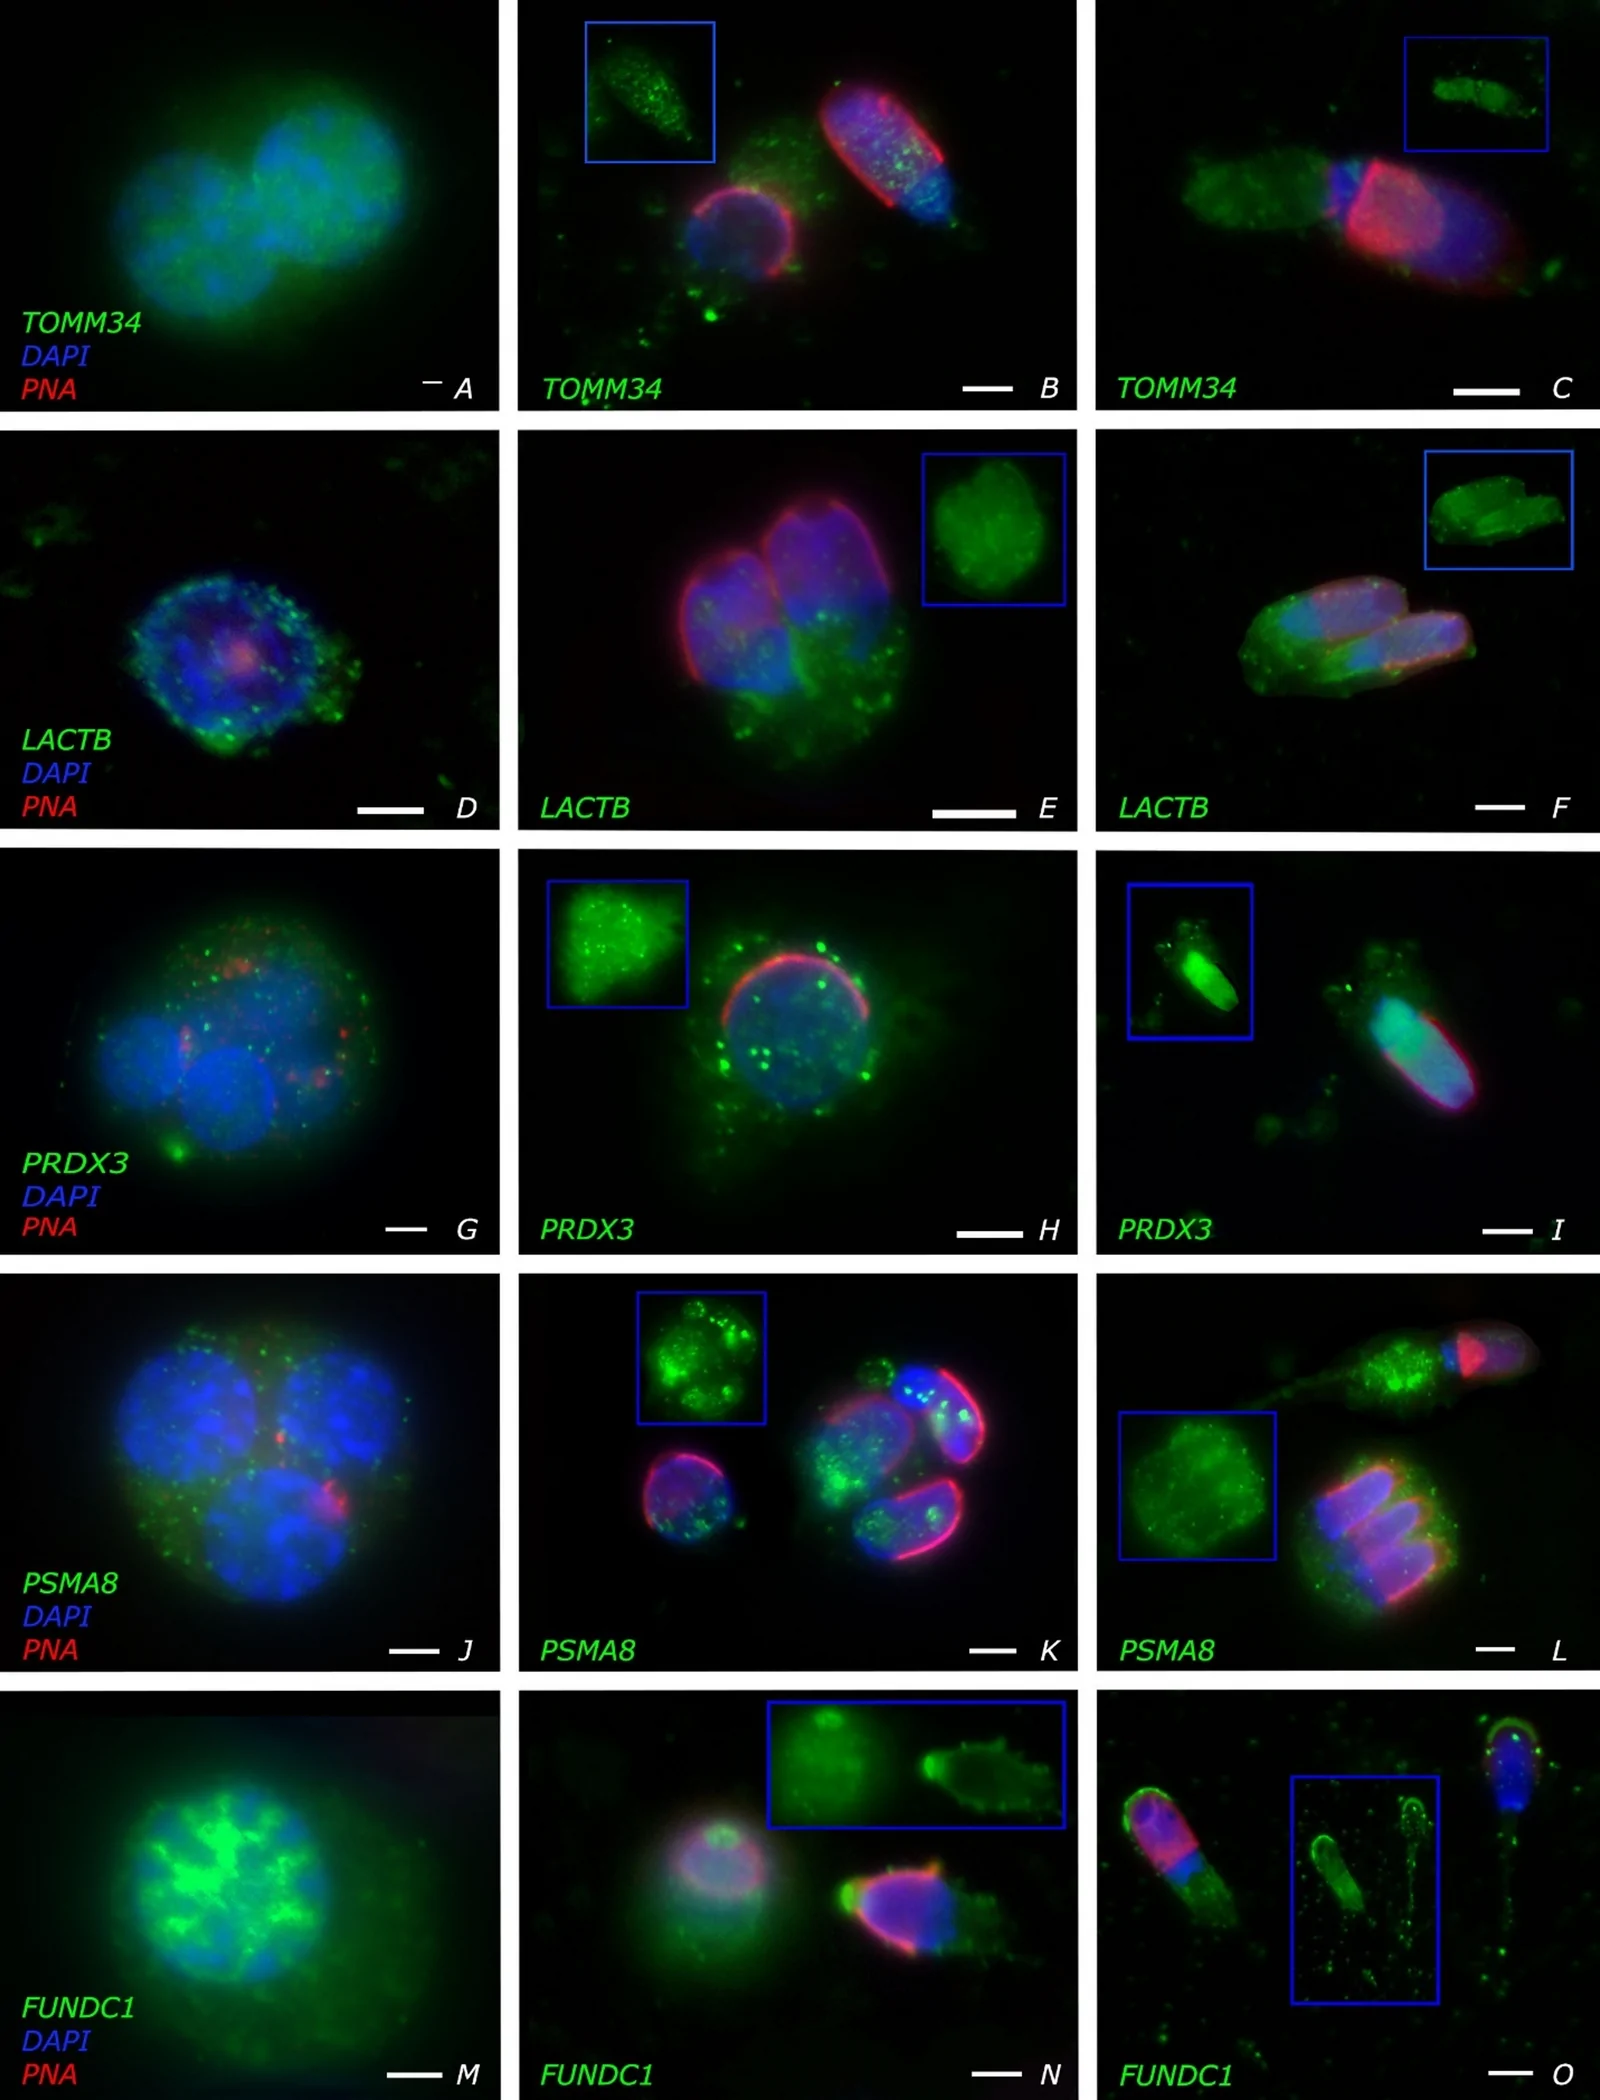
Immunocytochemistry of testicular germ cells provided visualization of the target proteins at various stages of spermatogenesis and steps of spermiogenesis. A The TOMM34 protein (green) was visualized within the cytoplasm of spermatocytes. B The signal persisted in the cytoplasm of round and early elongating spermatids, localizing faintly with the post-acrosomal region and developing connecting piece or centriole, as visible in the inset showing the TOMM34 signal alone. C As the spermatid elongation advanced, TOMM34 became weaker near the apical region, while stronger over the post-acrosomal region and cytoplasmic lobe/droplet and still present in the head. D The LACTB protein (green) was visualized within the cytoplasm of the spermatocytes. E The protein signal remained within the developing head and cytoplasm of the round spermatids. F In elongating spermatids, signals were localized to the outer acrosomal membrane, manchette, and the cytoplasmic lobe, as visible in the inset. G The PRDX3 protein (green) was visualized within the cytoplasm of the spermatocytes. H The signal intensified throughout the cytoplasm with the addition of granules/aggregates in round spermatids. I Through the elongation stages, PRDX3 can be seen in the head, with a stronger presentation in the post-acrosomal region. Protein signal decreased within the cytoplasmic lobes, as shown in the inset. J The PSMA8 protein (green) was visualized within the cytoplasm of the spermatocytes. The signal remained throughout the cytoplasm of round spermatids with the addition of granules. K There was a localization to the acrosomal membrane and post-acrosomal region. The PSMA8 can be visualized in the cytoplasm of elongating spermatids, with stronger signal on the outer edge of the acrosome (K, L). L In late-stage elongation and immature spermatozoa, there was a signal in the cytoplasm with an increase in signal intensity in the lobe/droplet and the developing mitochondrial sheath of the nascent tail. M The FUNDC1 protein (green) was visualized within the cytoplasm of the spermatocyte. The signal remained throughout the cytoplasm of round and early elongating spermatids. N The signal co-localized strongly with the acrosomal granule and outer acrosomal membrane. Through early and late elongation stages, FUNDC1 can be found within the acrosomal cap and cytoplasmic lobe. O The signal decreased overall as the spermatozoa completed elongation; FUNDC1 was visualized then over the mitochondrial sheath and tail and presented stronger to the acrosomal cap, as visible in the insets. Sperm acrosomes (red) were labeled with PNA-TRITC, DNA was counterstained with DAPI (blue). Scale bars = 5 μm
